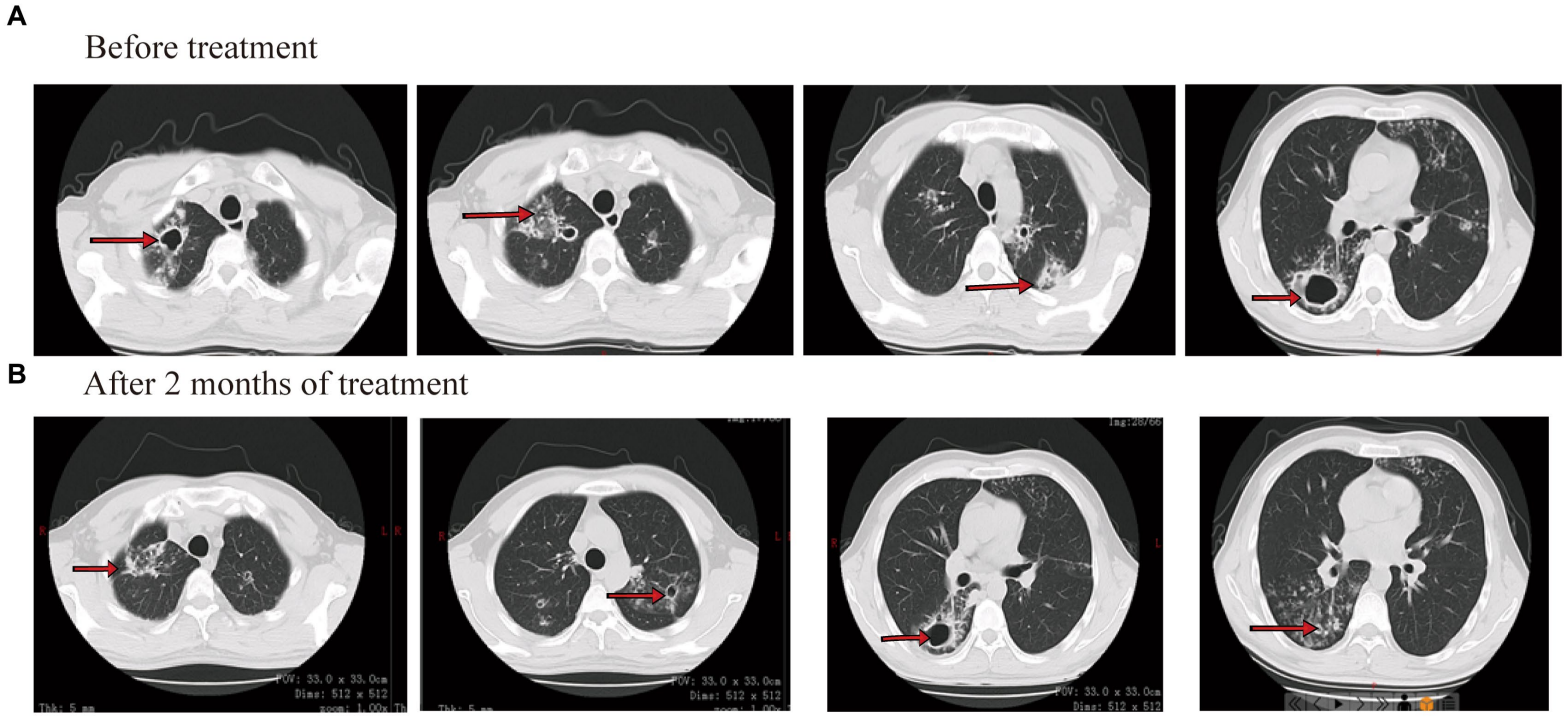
Chest CT scan images before and after treatment for case 2.
